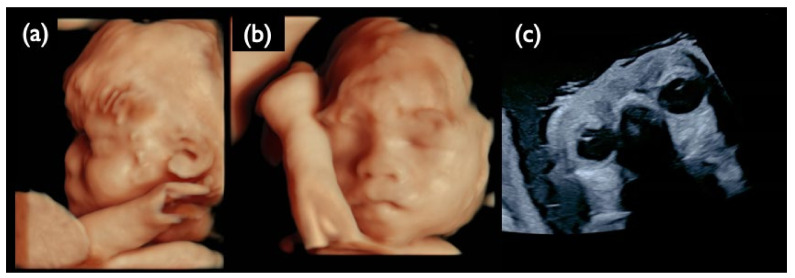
Ultrasound images at 30 weeks of gestation in the NC1 case. (a) 3D ultrasound image of the fetal profile. (b) 3D ultrasound image of the frontal face of the fetus. (c) Ultrasound image of fetal eye sand lenses on both sides. All images show that the fetus is hypertrichotic and has no ocular lesions at this gestational age.
